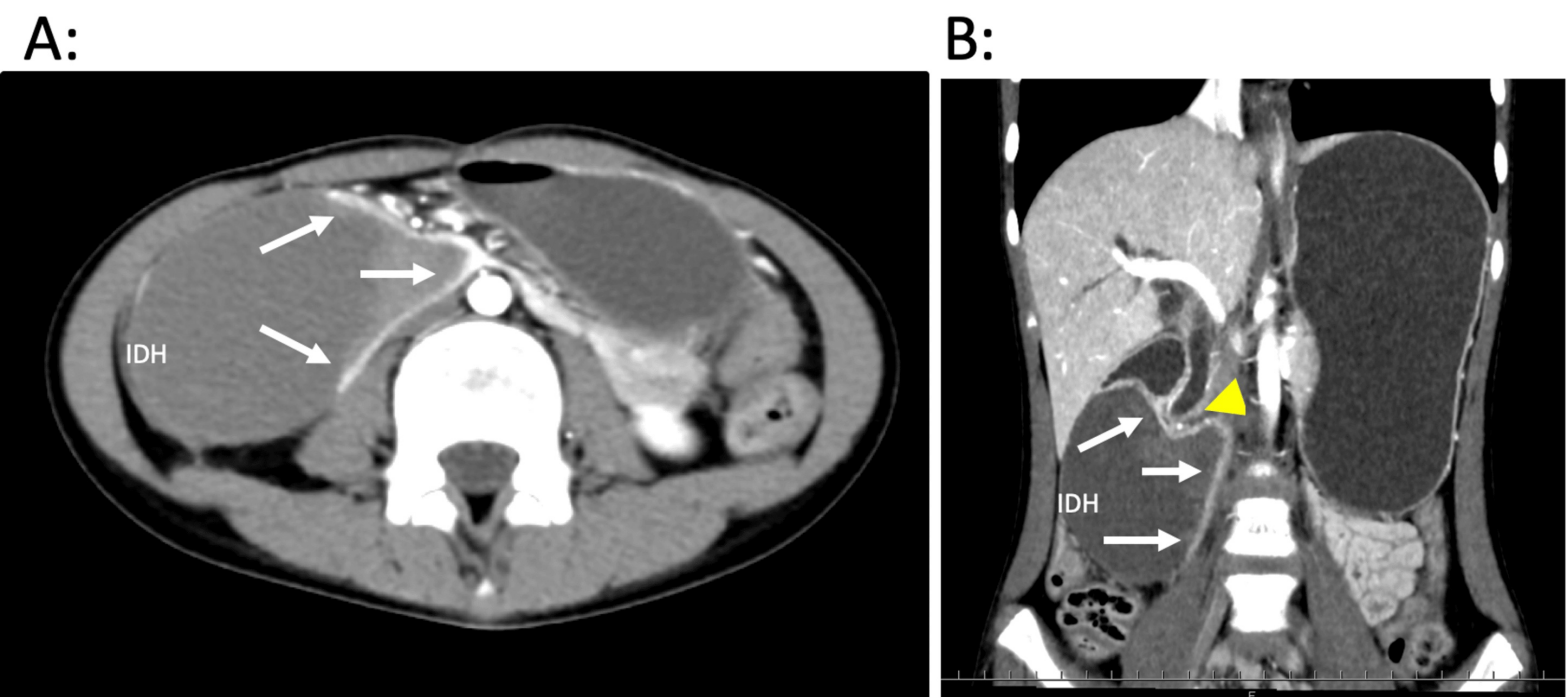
Contrast-enhanced abdominal computed tomography in (A) axial and (B) coronal views. The intramural duodenal hematoma, measuring 7.0 cm × 4.5 cm, compressed and obstructed the duodenum (arrow) and also compressed the bile duct (arrowhead).
